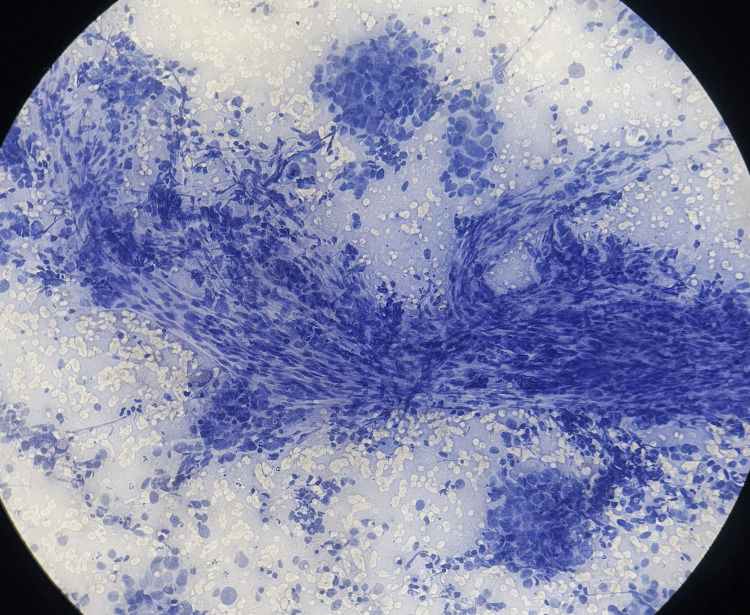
USG-guided FNAC of HCC showing transgressing of capillaries. USG: Ultrasonography; FNAC: Fine-needle aspiration cytology; HCC: Hepatocellular carcinoma
